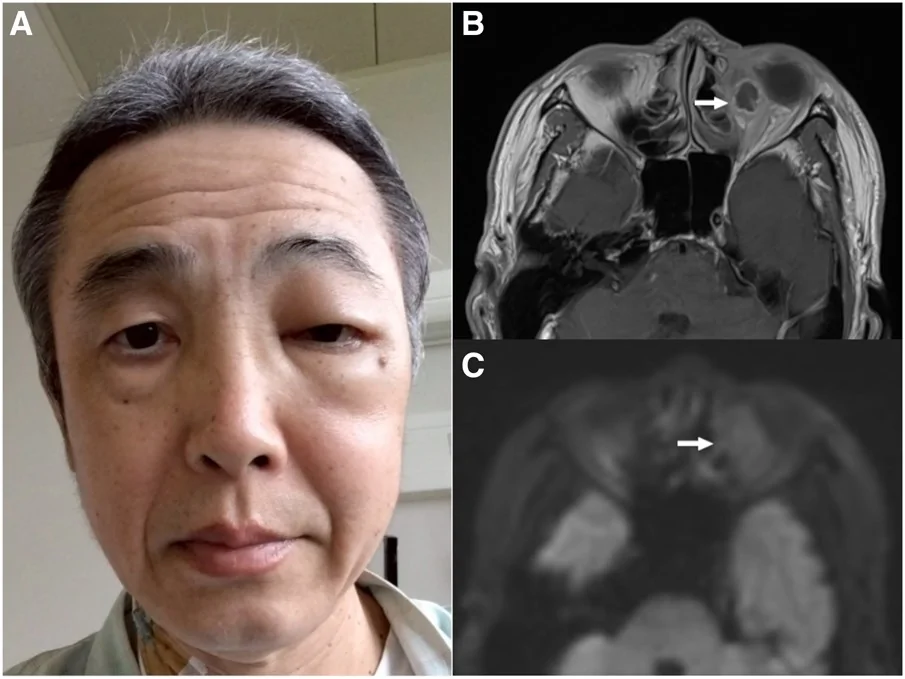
(A) Frontal photograph obtained on day 12 after CAR T-cell infusion shows painless left periorbital swelling. Magnetic resonance imaging obtained on the same day shows a heterogeneous ring-enhancing lesion in the left inferior orbit at the site of prior lymphoma involvement (B), while corresponding diffusion-weighted magnetic resonance imaging shows no diffusion restriction within the lesion (C).
